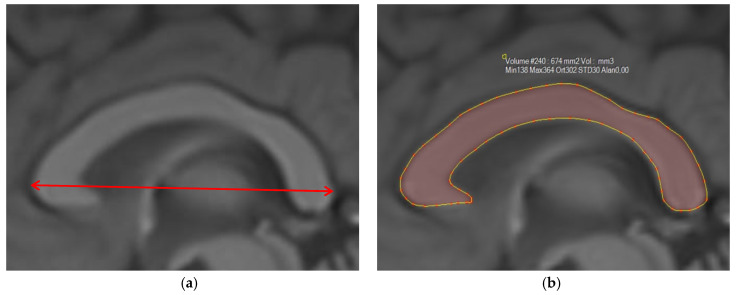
The midsagittal T1W MRI image shows (a) the anterior–posterior diameter and (b) the area of the corpus callosum.
